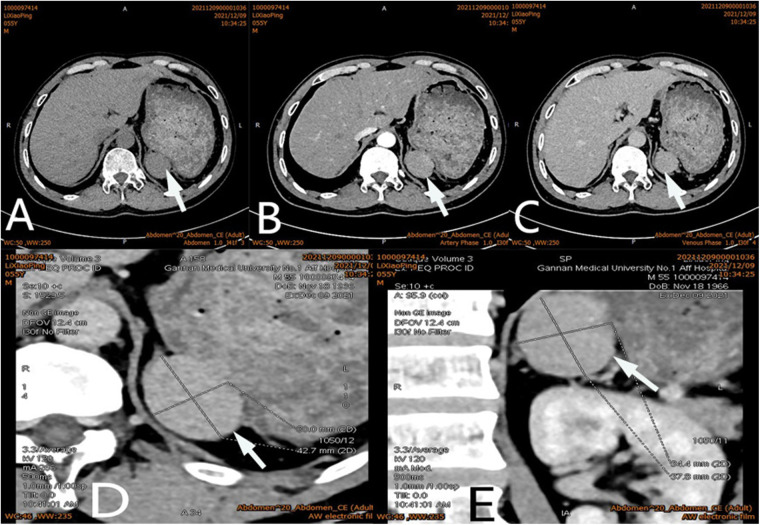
Ct scan of a mass (arrow) in the left adrenal region. The CT value of plain scanning which the internal density was heterogeneity (A), and enhanced scanning presented moderate enhancement (B), the delayed phase of the mass (C). Coronal image of the mass (D). Sagittal image of the mass (E).
